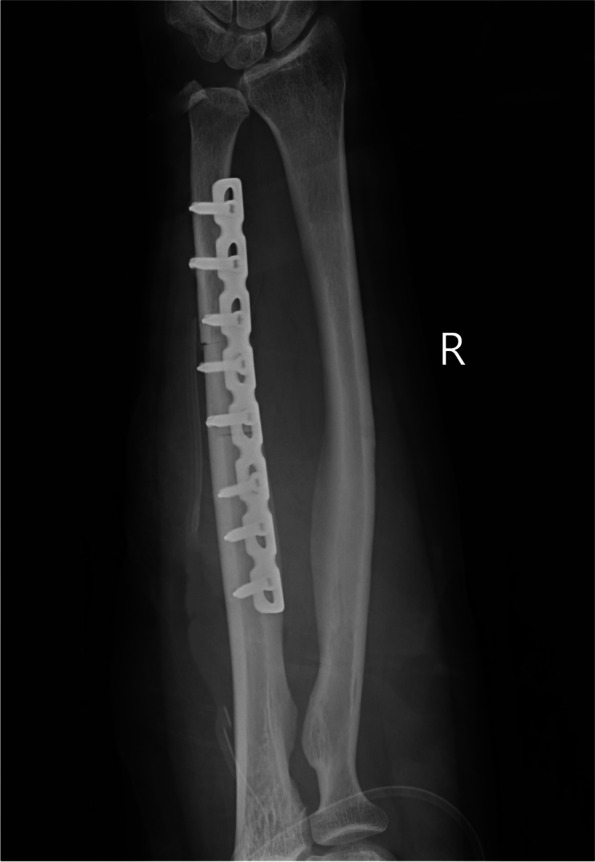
Postoperative radiograph of diaphyseal step-cut USO
